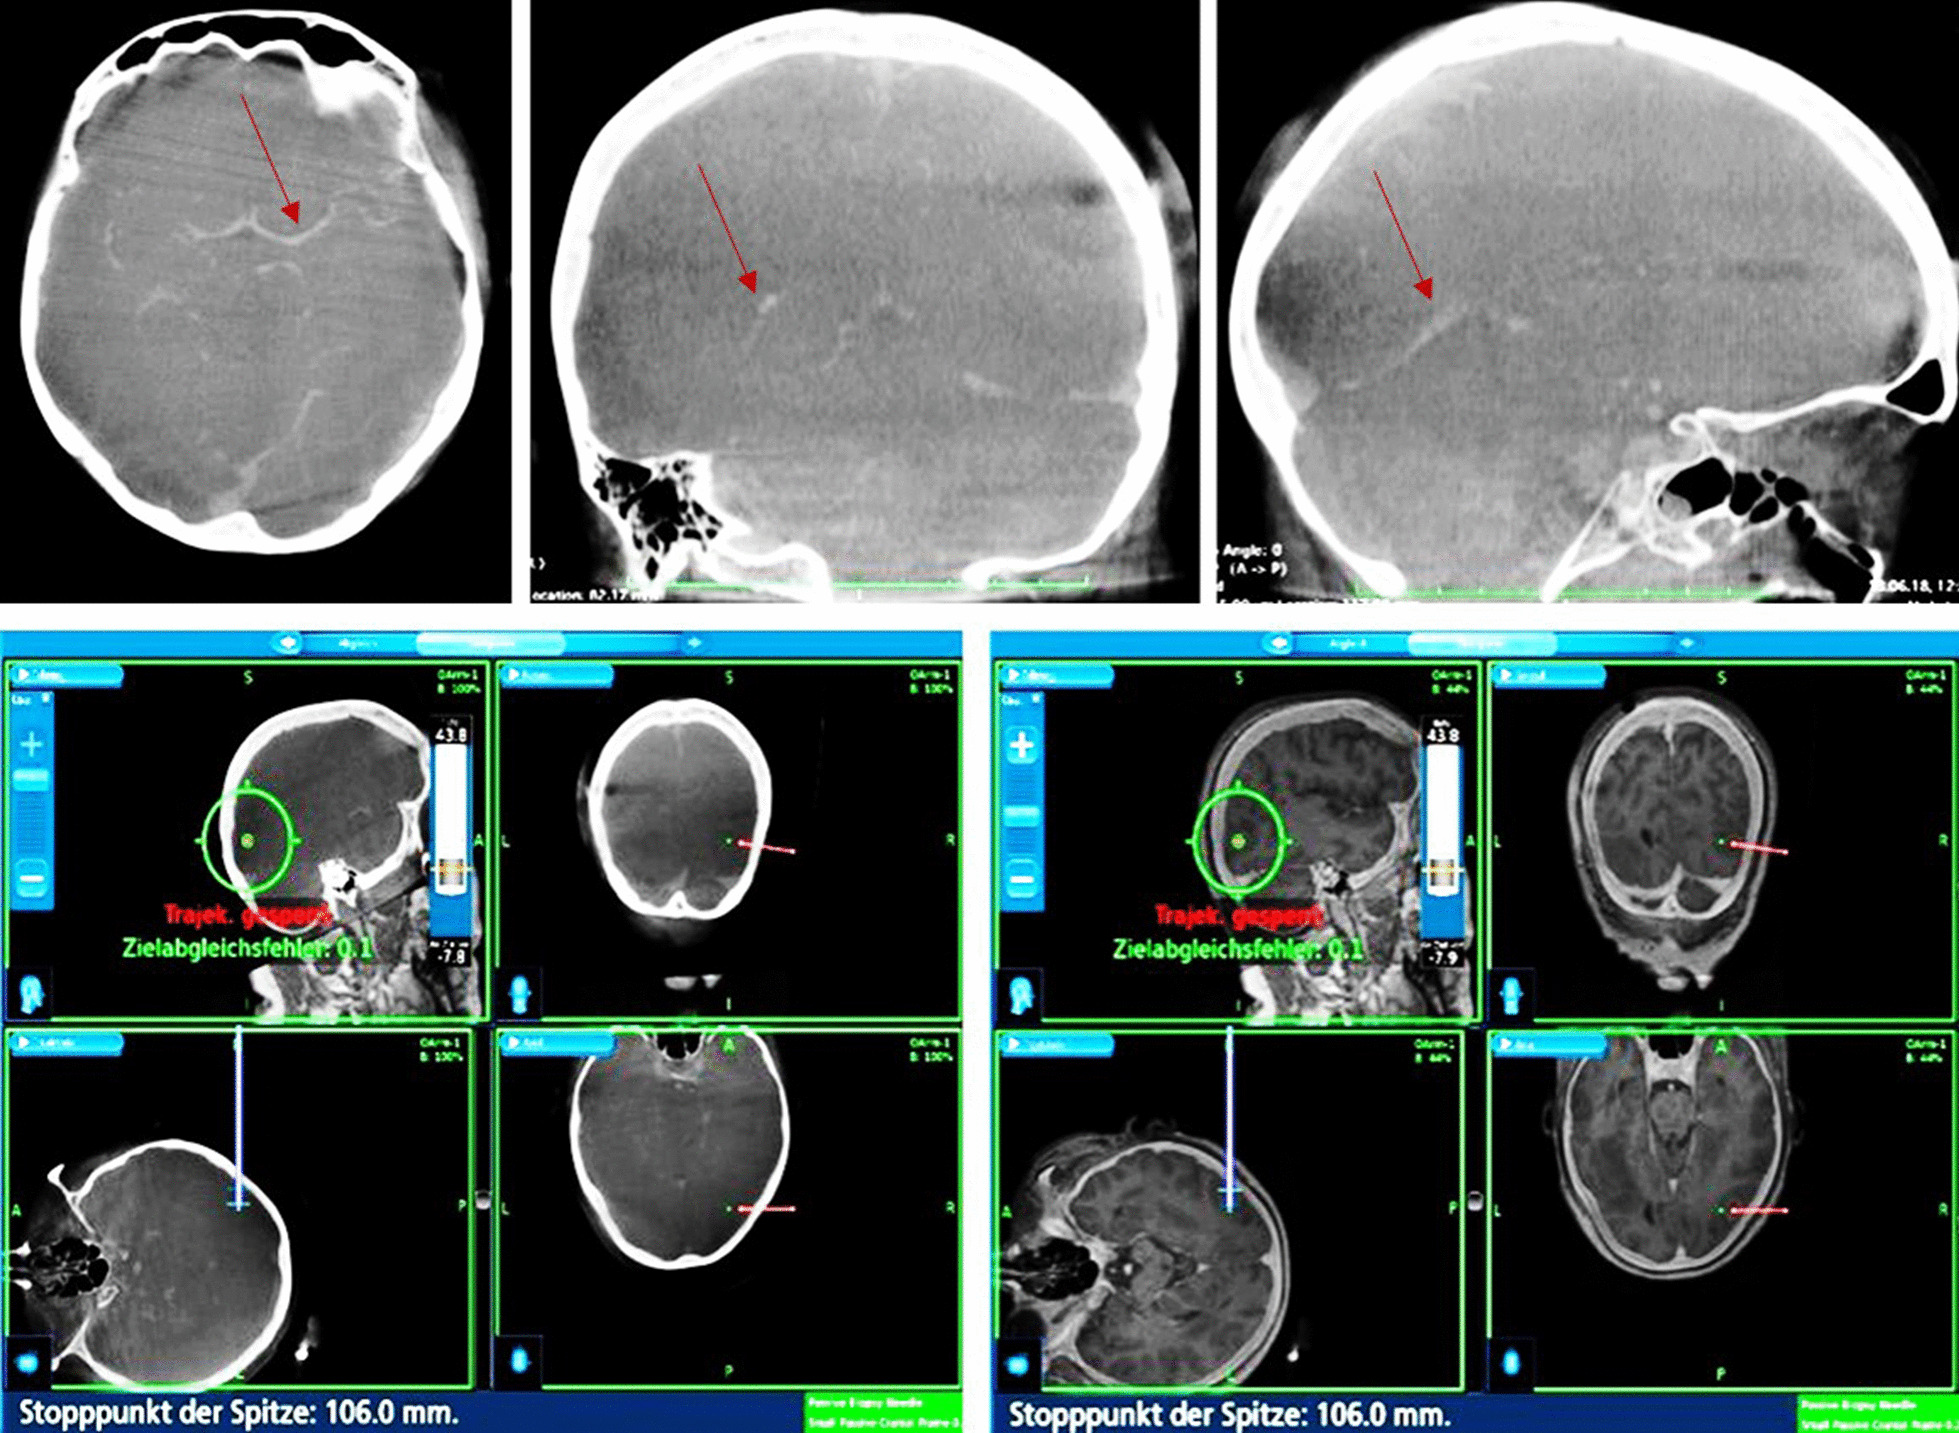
a–c Acquired images after 3-D rotational fluoroscopy scan with a delay of 12 s after the beginning of the contrast infusion. Visibility of the bony structures and vessel anatomy indicated by red arrows. d, e DICOM data sets transferation to the MedTronic Stealth Station S7. Merging with standard MRI-MPRage data set. Example for “bone—overlay” (d) and 50% MRI—overlay (e)
